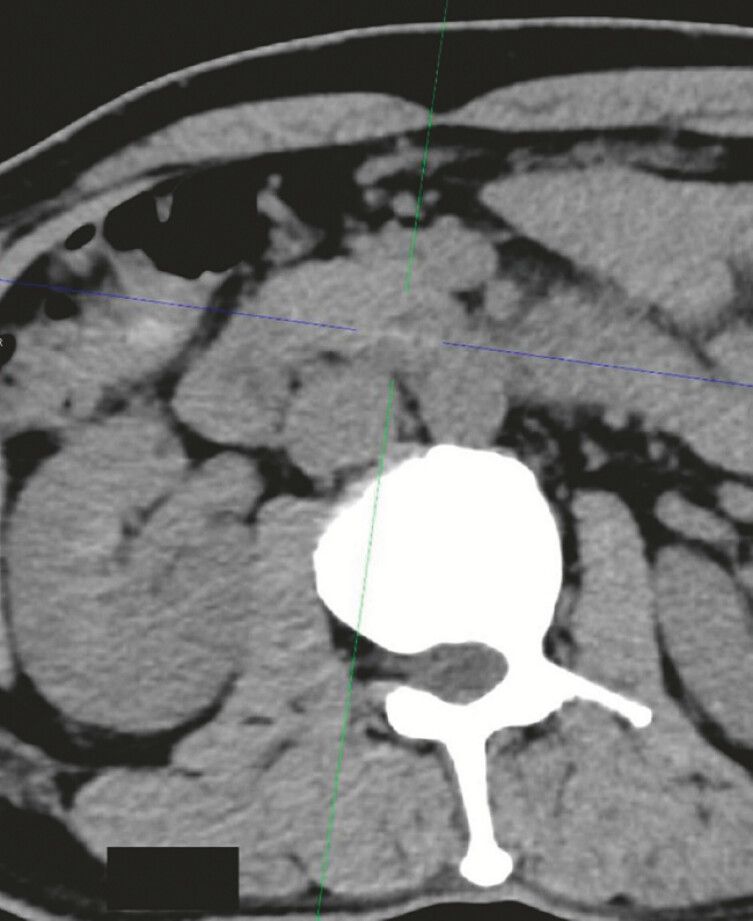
Computed tomography image showing an elevated fat density surrounding the proximal segment of the superior mesenteric artery, along with a speckled gas density shadow, suggestive of potential duodenal perforation.
